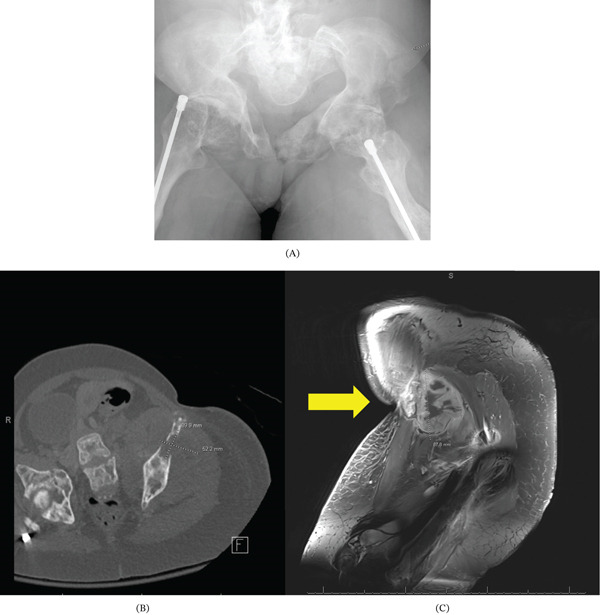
(A) XR pelvis demonstrating a nondisplaced fracture of the iliac wing with bony remodeling. (B, C) CT lower extremity and MRI pelvis demonstrating a heterogeneously enhancing extraosseous mass arising from the ilium.
